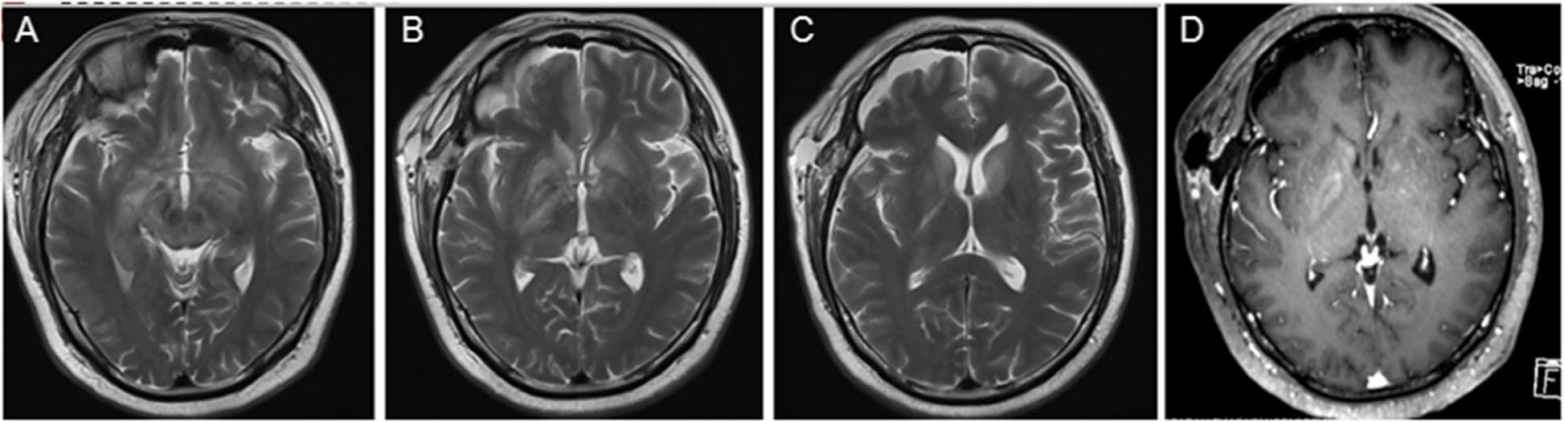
MRI at discharge. a-c T2-weighted sequence, the lesions in the bilateral basal ganglia, thalamus, brainstem, hippocampus, and temporal lobe were significantly reduced compared to those in Fig. 1. d T1 weighted image after contrast: the enhancement is reduced
